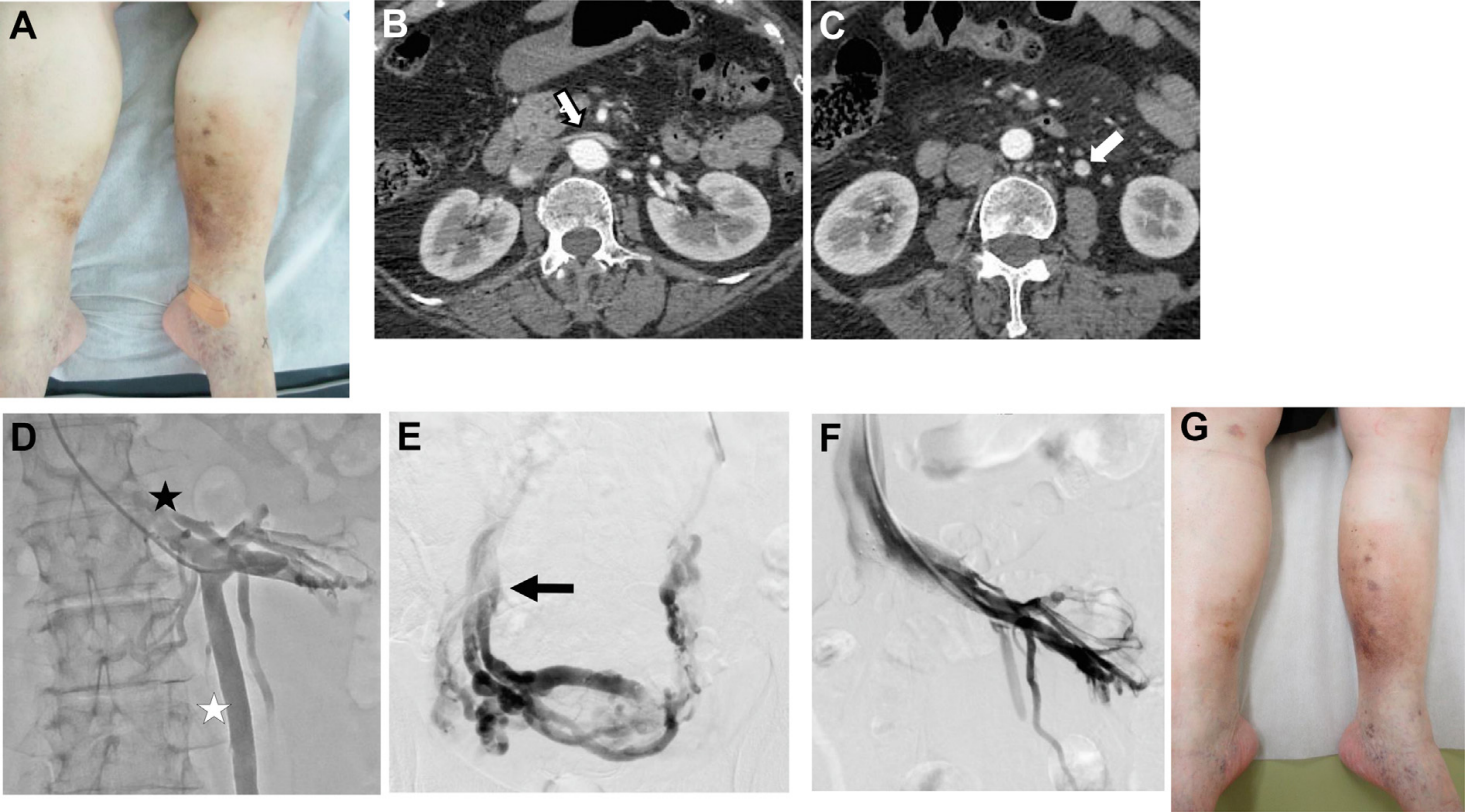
Case 2. (A) Preoperative photograph of the patient showing swelling, dermatitis, and hyperpigmentation of both lower extremities, and a bleeding ulcer on her left leg. (B) Computed tomography angiography (CTA) showing the left renal vein (LRV) compression between the superior mesenteric artery and the aorta (white arrow). (C) Early opacification of the left gonadal vein (LGV) (white arrow). (D) Venogram shows compression of the LRV (black star) and the collateral pathway (LGV reflux) (white star). (E) Selective left gonadal venography demonstrates reflux in the LGV associated with pelvic varices draining into the right common iliac vein (CIV) (black arrow). (F) After LRV stenting, the collateral pathway was reduced. (G) One-month postoperative photograph showing improvement in lower extremity symptoms.
